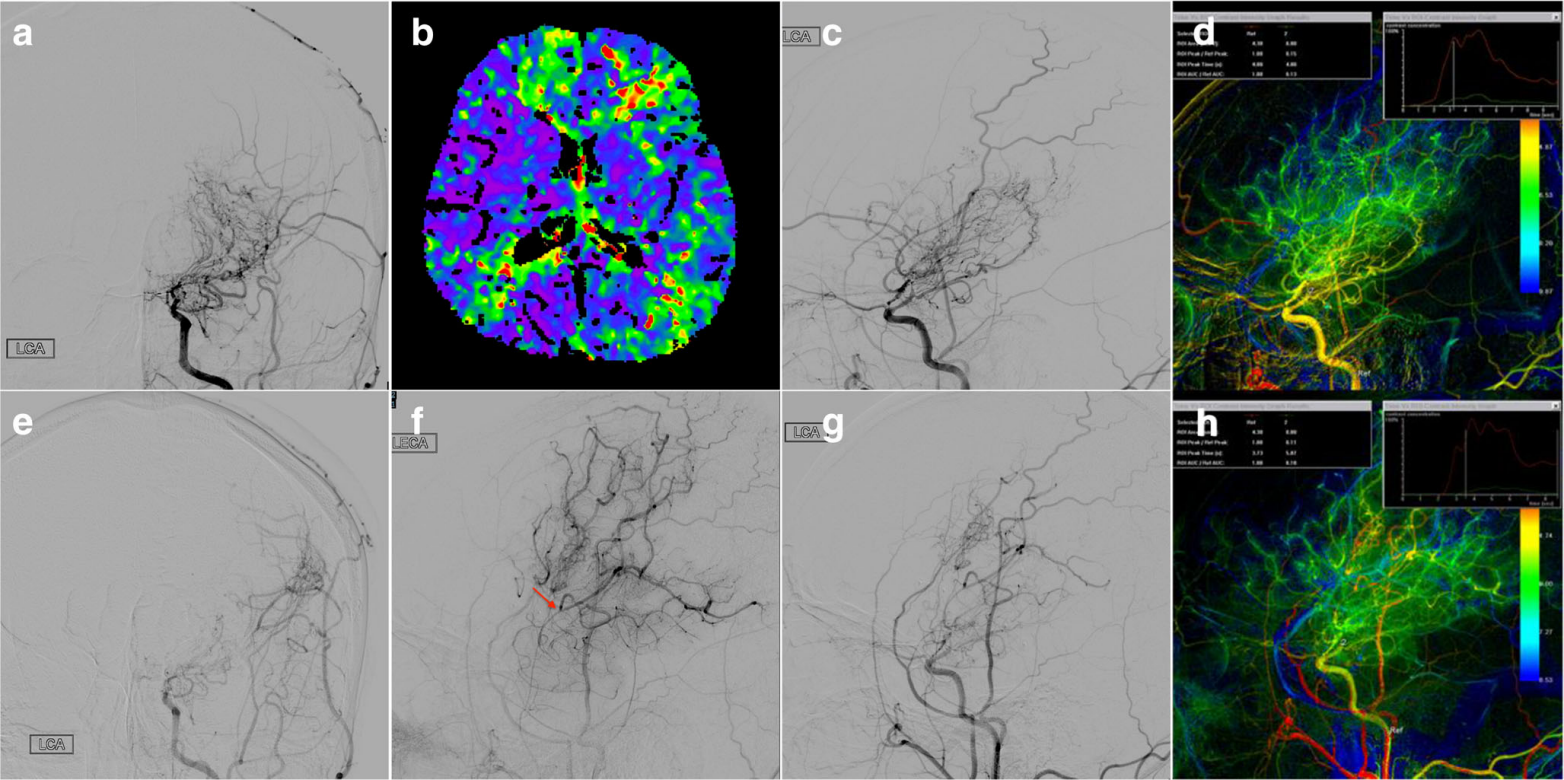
Preoperative digital subtraction angiography (DSA) of the left hemisphere showed Suzuki stage III moyamoya disease (MMD) and large amount of moyamoya vessels (a). Cerebral blood flow (CBF) of computed tomography perfusion (CTP) showed poor perfusion in the left middle cerebral artery (MCA) territories, especially in the left frontal lobe (b). The preoperative blood flow in the internal carotid artery (ICA) was fast, and the ICA-MTT was 0 s (c, d). Six months later, the moyamoya vessels of the left hemisphere were significantly reduced (a vs e). Good angiogenesis was observed and evaluated as Matsushima grade A (anastomotic site: red arrow) (f). The postoperative blood flow in the ICA was significantly prolonged to 1.34 s (g, h)
